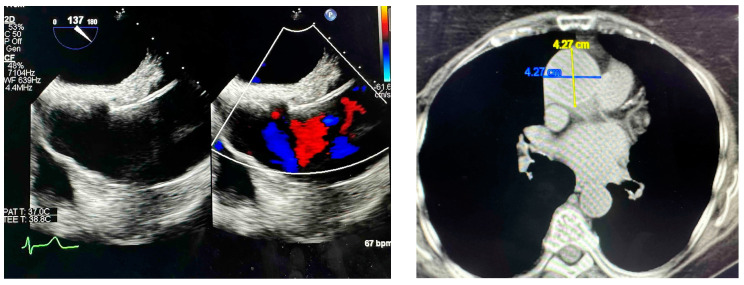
(Left Panel): TEE imaging demonstrating a 4.3 cm ascending aortic aneurysm in subject 2. (Right Panel): CTA measuring an ascending aortic aneurysm of 4.3 cm × 4.3 cm in subject 2.
